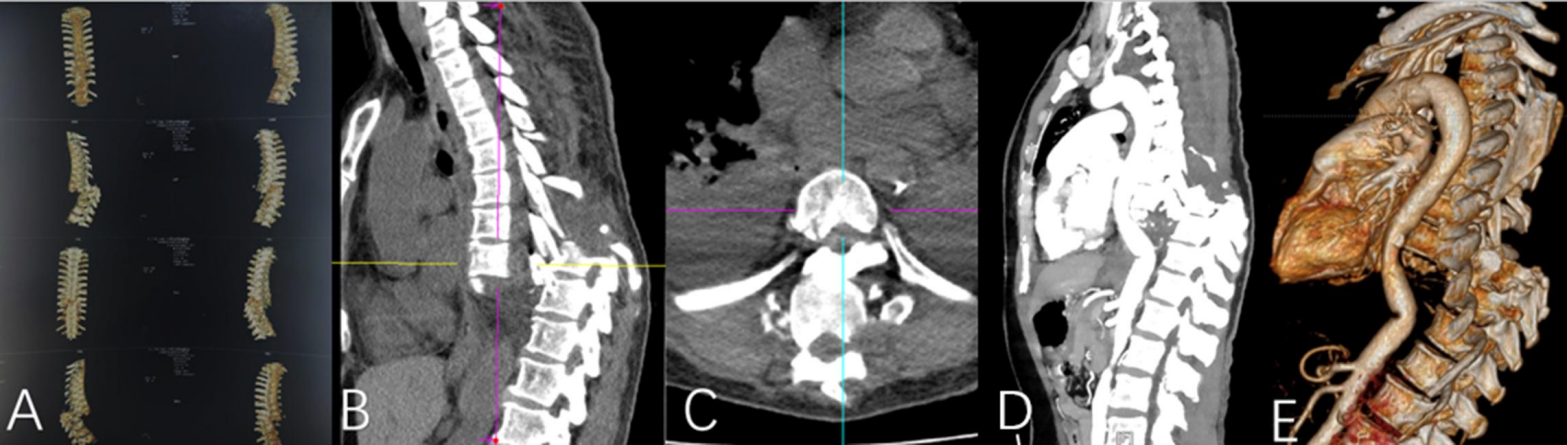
Thoracic spine (A–E) computed tomography (CT) images clearly demonstrate the severe spinal injury and adjacent vascular relationships at the T8–T9 level. A complete fracture-dislocation is observed between the T8 and T9 vertebral bodies. The distal fracture fragment is displaced posteriorly by approximately the combined width of one vertebral body and its posterior elements, accompanied by superior displacement of about one vertebral body height in the vertical plane. Complete fractures are evident in the posterior elements at the corresponding level, including the pedicles and facet joints. Additionally, free bone fragments are visible below the proximal fracture segment.
